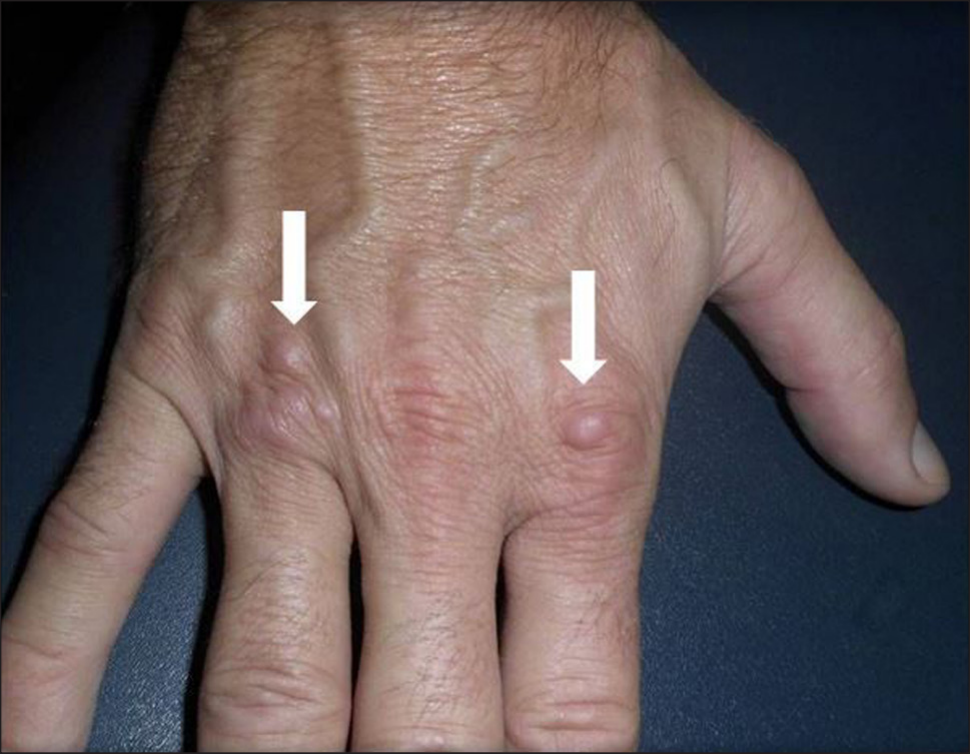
Ultrasound (US) showed two flat dome shaped hypoechogenic lesions at the subcutaneous aspect of MCP joints 2 and 4 (Figure 2, arrows).
